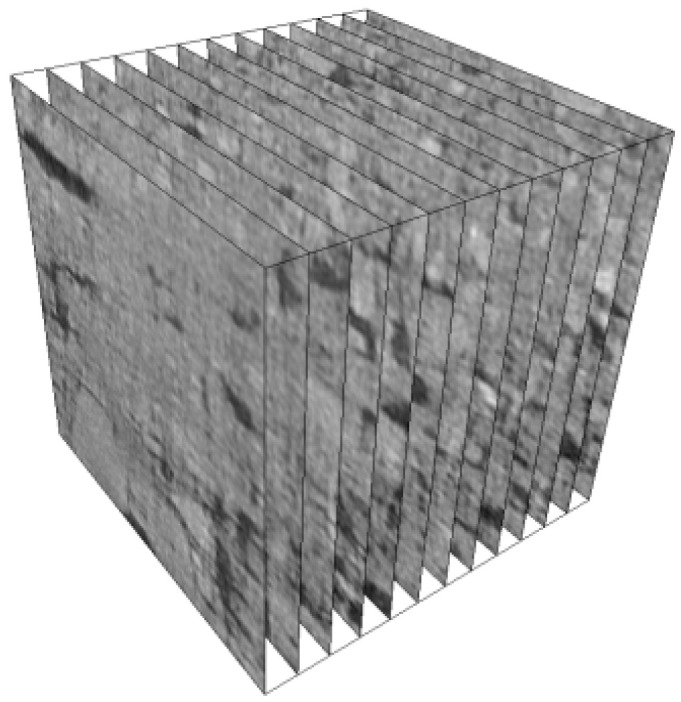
Representative slices of X-ray CT images of a U-GRS sample.
